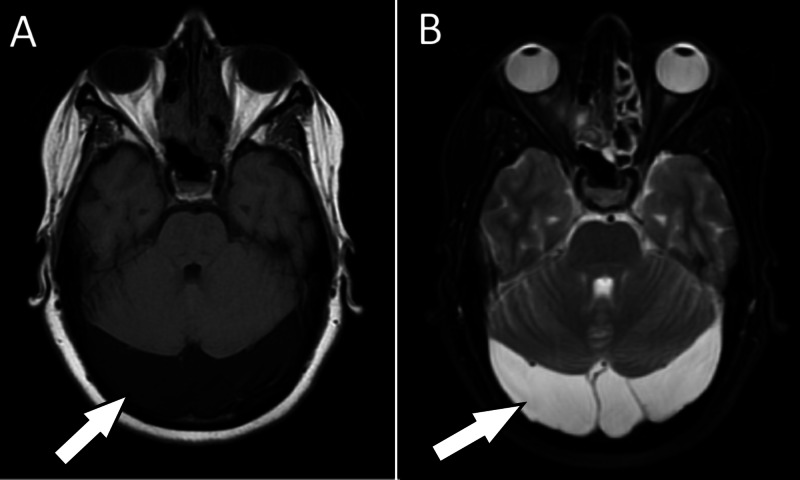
MRI of the brain without contrast shows bilateral retrocerebellar arachnoid cysts with mild mass effect on the cerebellum, which appear (A) hypointense on T1-weighted imaging and (B) hyperintense on T2-weighted imaging.
